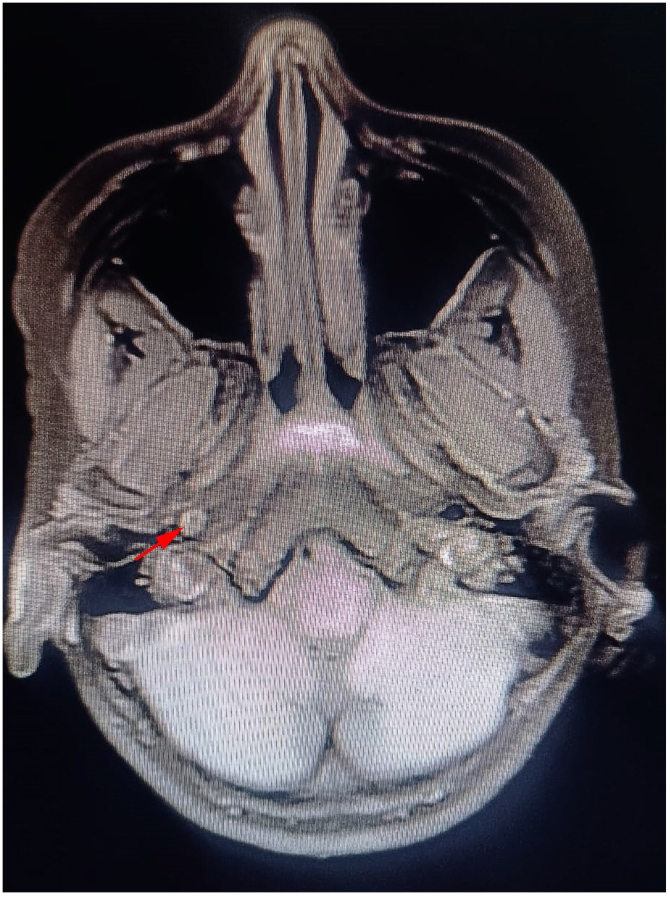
Angio-CT scan of the supra-aortic trunks showed a narrowed right internal carotid artery, measuring 2 mm in diameter.
